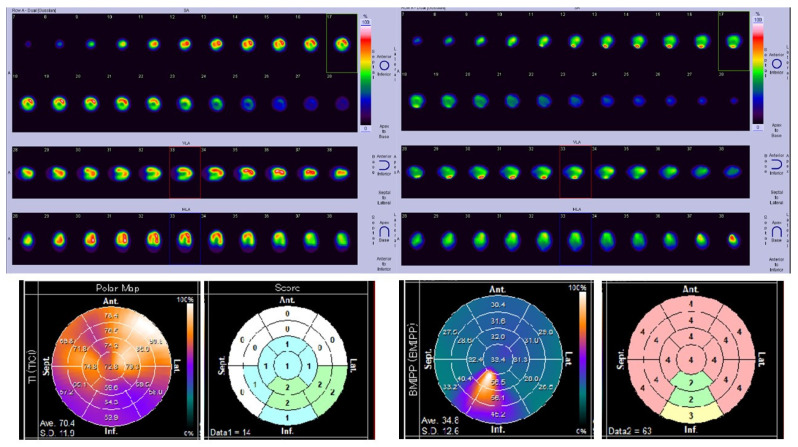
SPECT images, polar maps, and Heart Risk View-S scores for Case G. Left top: SPECT images of ^201^Tl perfusion scintigraphy. Left bottom: polar map and scores of ^201^Tl. Right top: SPECT images of ^123^I-BMIPP metabolism scintigraphy. Right bottom: polar map and scores of ^123^I-BMIPP.
